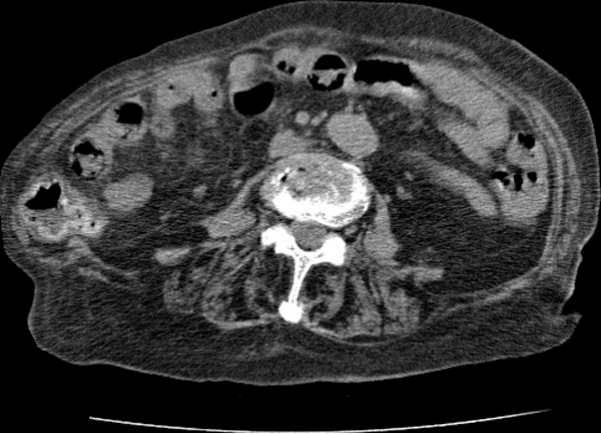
Axial CT scan obtained with IV contrast material in 84-year-old female subject diagnosed with ischemia.
